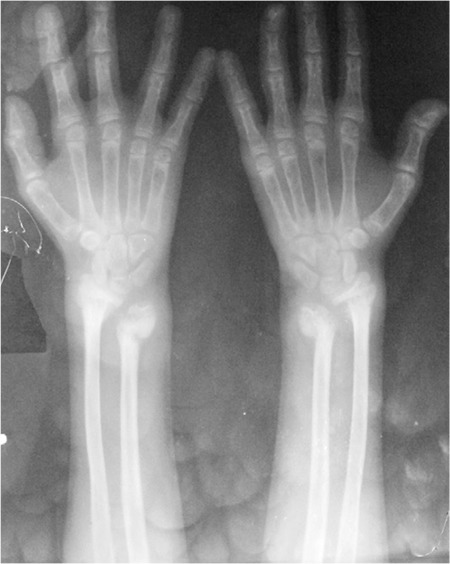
Radiograph of the hands and forearms showing generalized osteopenia, widening of the distal ends of the radius and ulna along with cupping, fraying and splaying of the metaphyses
